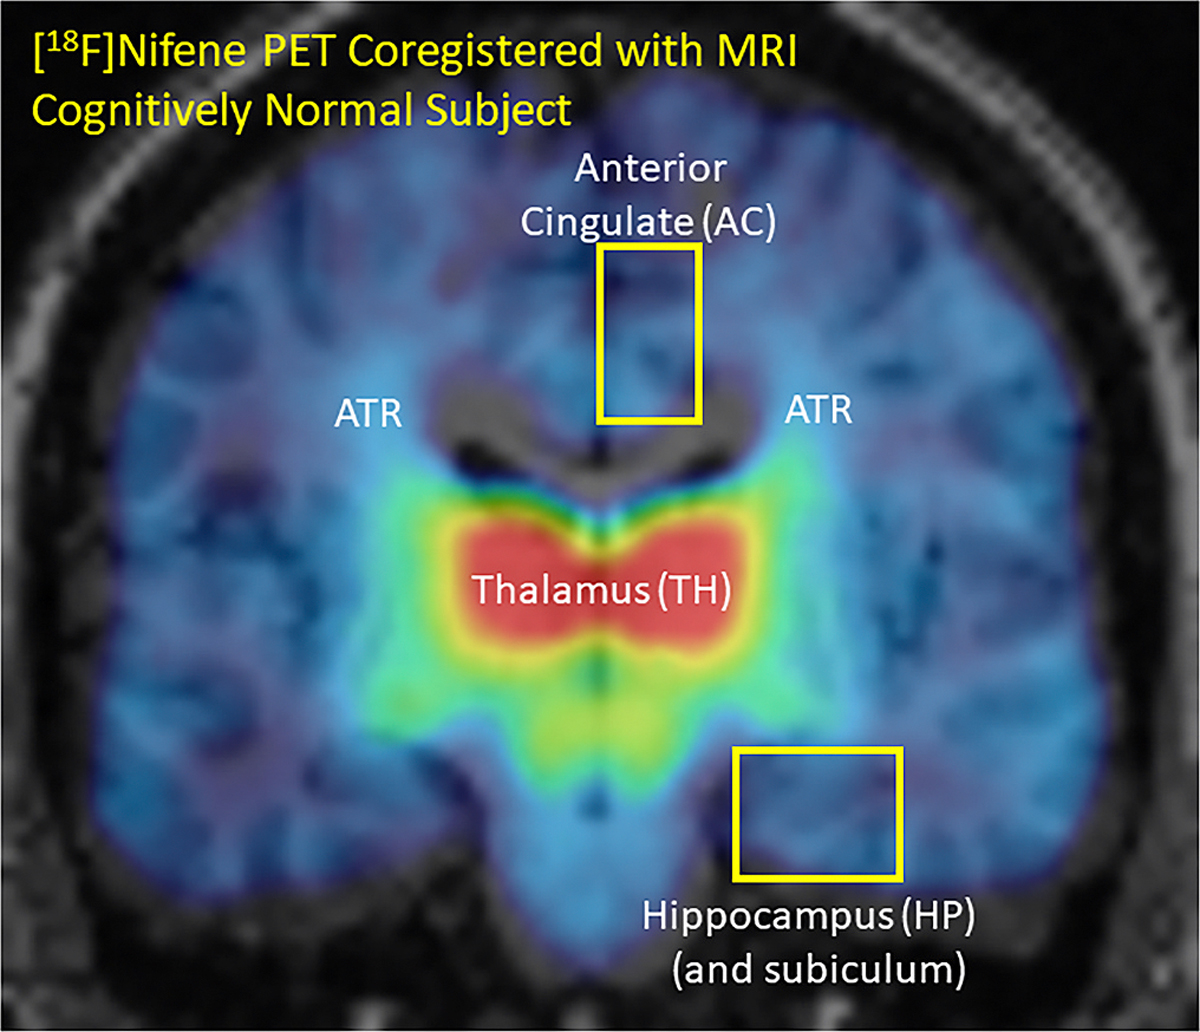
[^18^F]Nifene for imaging human α4β2* nAChRs: Coronal brain slice of cognitively normal subject showing binding of [^18^F]nifene coregistered with the subject’s MRI (Mukherjee et al., 2018). High binding of [^18^F]nifene is seen in the thalamus (red), white matter anterior thalamic radiations (ATR in green) project to the various cortical regions (blue). [^18^F]Nifene binding in anterior cingulate and HP (yellow boxes) is of particular interest in AD.
